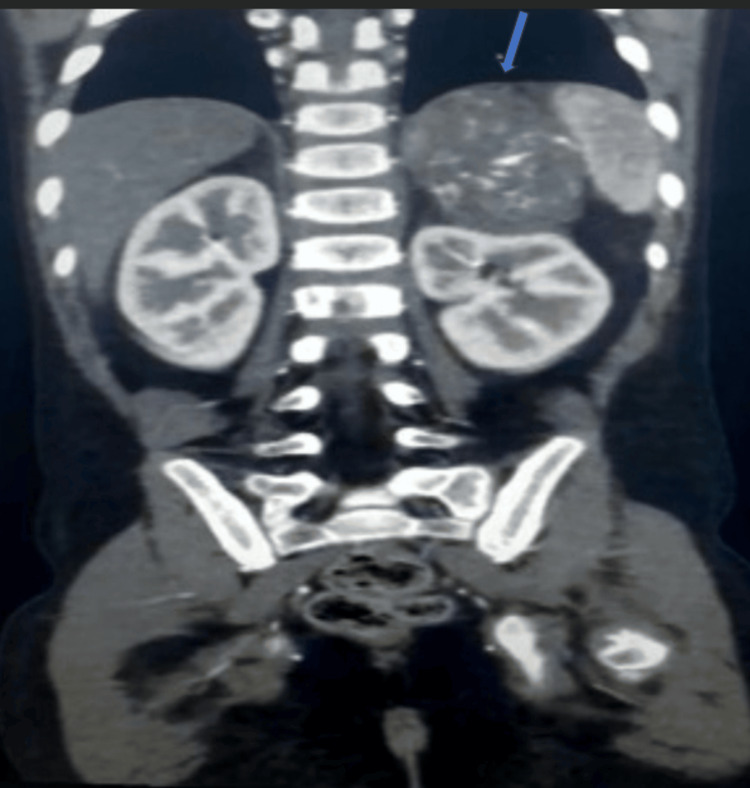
Computed tomography angiography (CTA) scan of the abdomen showing a large, heterogeneously enhancing mass (arrow) arising from the upper pole of the left kidney, consistent with an adrenal origin.
